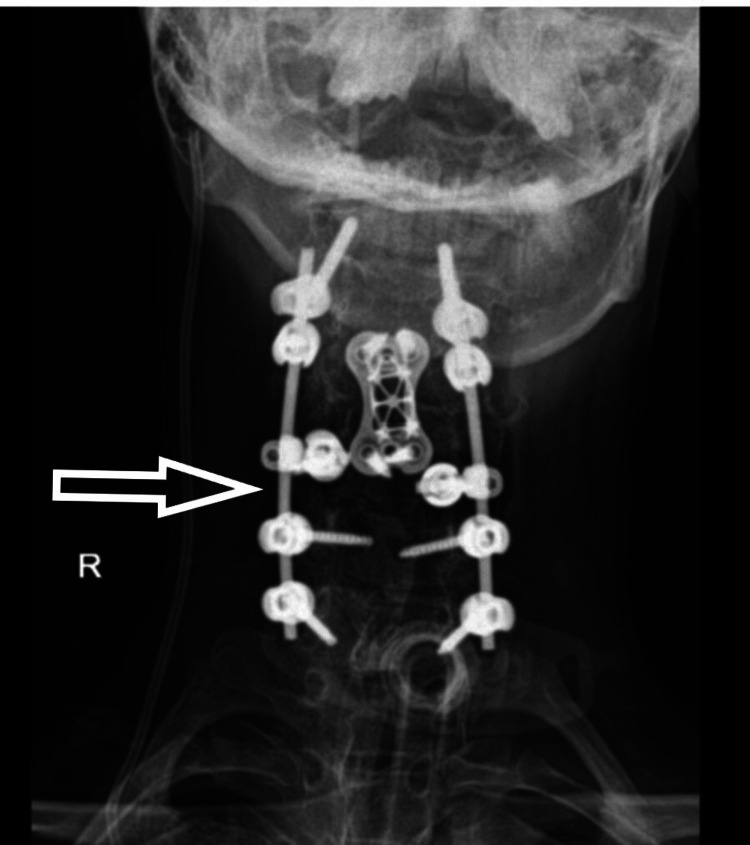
Postoperative X-ray - image 1
